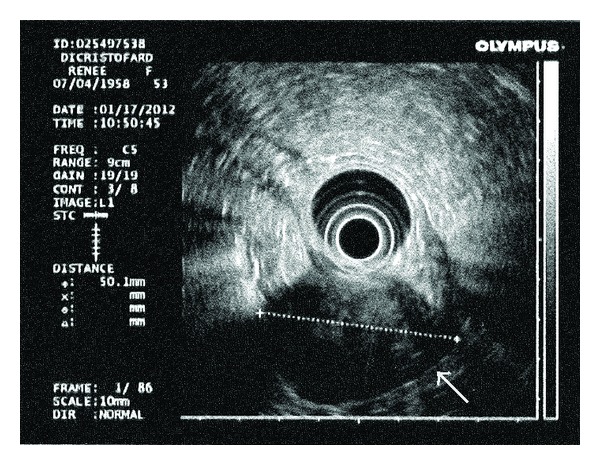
Endoscopic ultrasonogram: a 5 cm hypoechoic inhomogeneous mass lesion with calcification is seen. The mass appears to arise from the muscularis propria.
